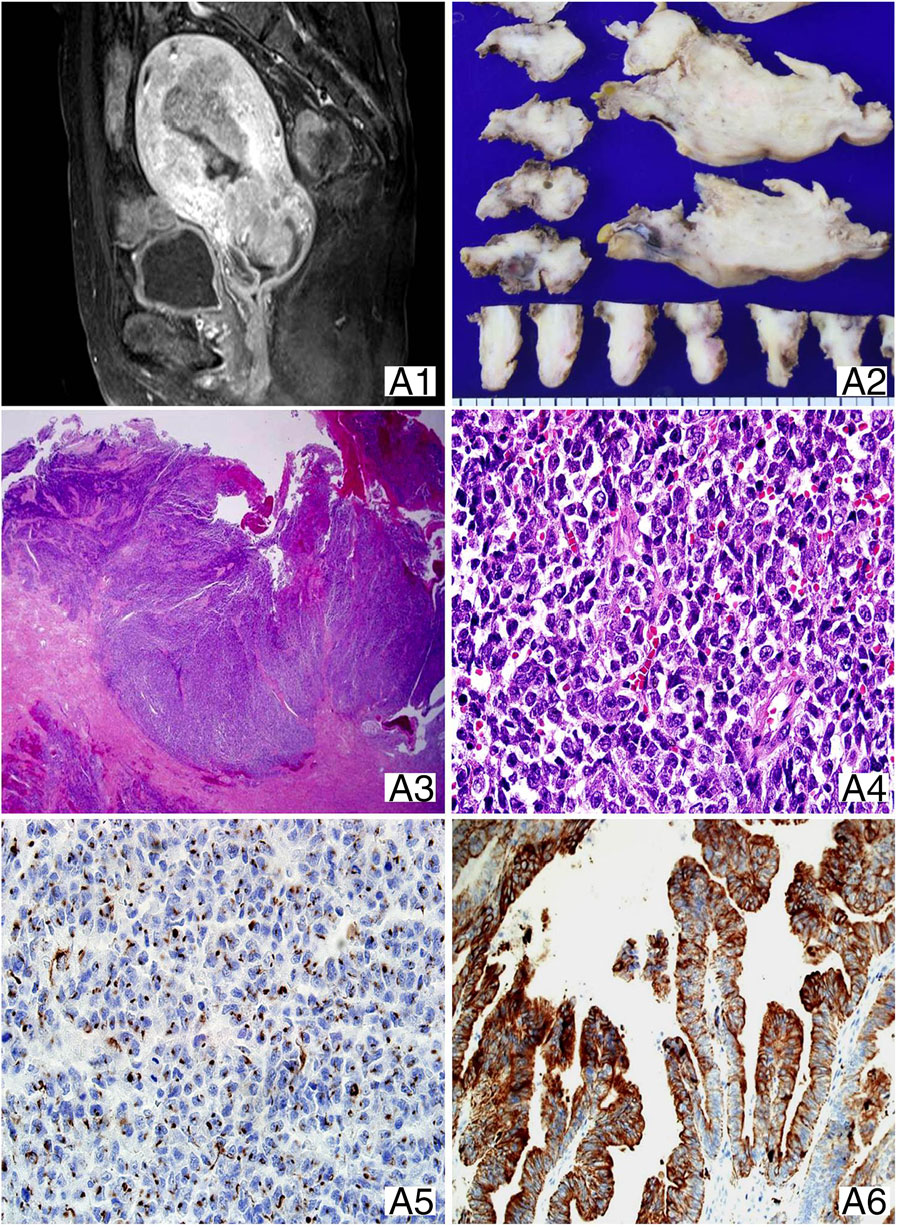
Case 1. (*A1*) Sagittal contrast-enhanced T1-weighted MRI shows heterogeneously enhanced mass filling the endometrial cavity with cervical stromal invasion. (*A2*) The tumor shows white infiltrative lesion with necrosis involving lower uterine segment and uterine body, extending to cervix. The UC component (*A3*, ×2.5) infiltrates the full thickness of the lower uterine segment and arranged in patternless with extensive necrosis and hemorrhage. The tumor (*A4*, ×400) comprises monotonous cells with moderate pleomorphism, prominent nucleoli, and high mitotic rates. Cytokeratin 8/18 (CK8/18) expressed as perinuclear dots in the UC component (*A5*, ×400) and showed diffuse cytoplasmic expression in differentiated component (*A6*, ×200)
